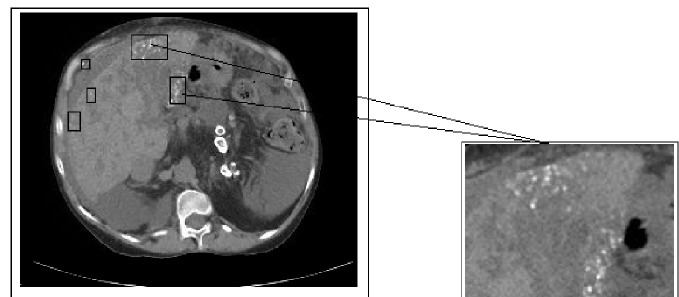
Abdominal CT scan showing multiple cystic hypo-densities with calcification in liver parenchyma.
